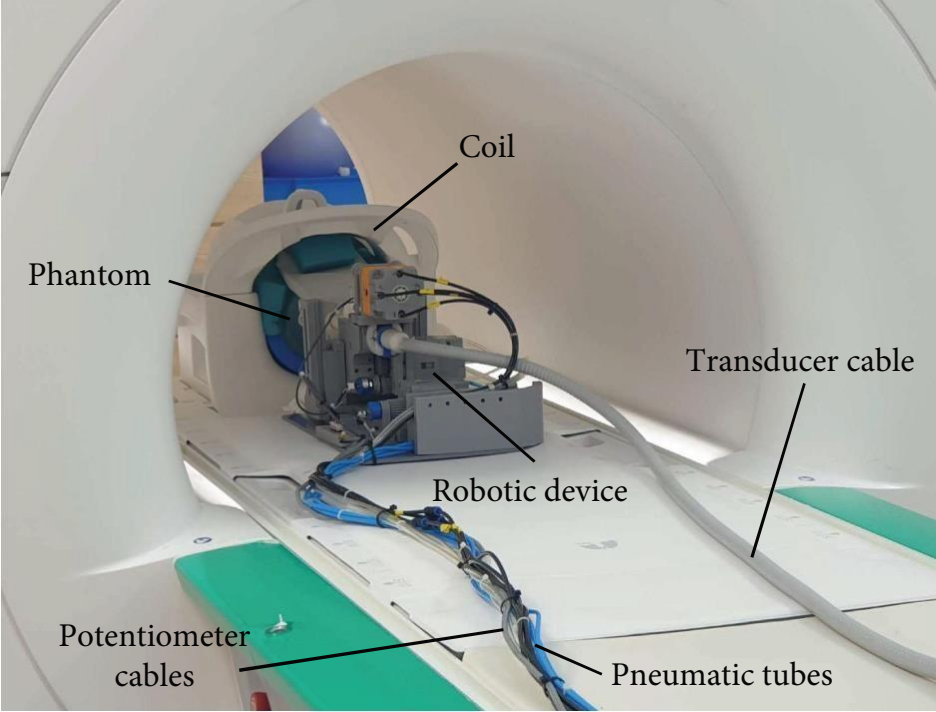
Experimental setup in the MRI scanner for assessing the MRI compatibility of the robotic device.
